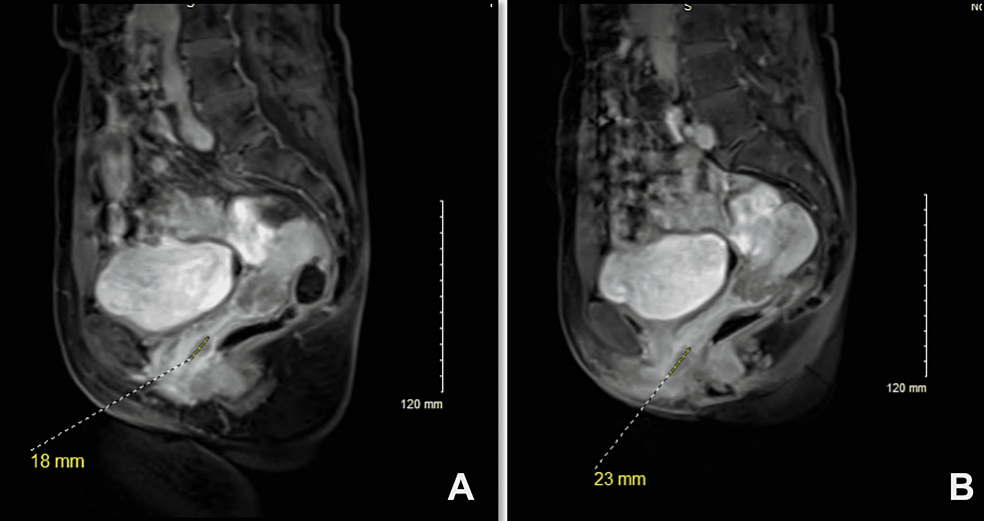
MRI of the pelvis A: The tumor is bilobed with a component of this tumor within the middle third of the vagina measuring 18 mm, B: There is a 2.3 cm mass involving the lower one-third of the vagina causing mass effect on and likely invading the distal female urethra
